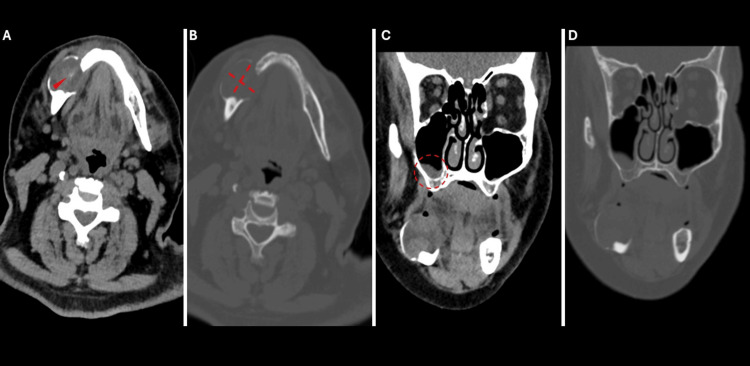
CT Imaging of the Right Mandibular Expansile Mass Non-contrast CT images of a rounded, heterogeneous, expansile mass in the right mandibular body measuring 3 x 5.3 cm: (a) Soft tissue window, transverse plane: The mass contains a small hyperdense focus, likely representing a blood vessel or hemorrhage. (b) Bone window, transverse plane: The mass demonstrates cortical and bony structure destruction. (c) Soft tissue window, coronal plane: The mass is in direct contact with the mylohyoid and platysma muscles. A retention cyst is observed in the right maxillary sinus, along with mucosal thickening in the left ethmoid cells. (d) Bone window, coronal plane: Additional visualization of the mass and its bony involvement.
